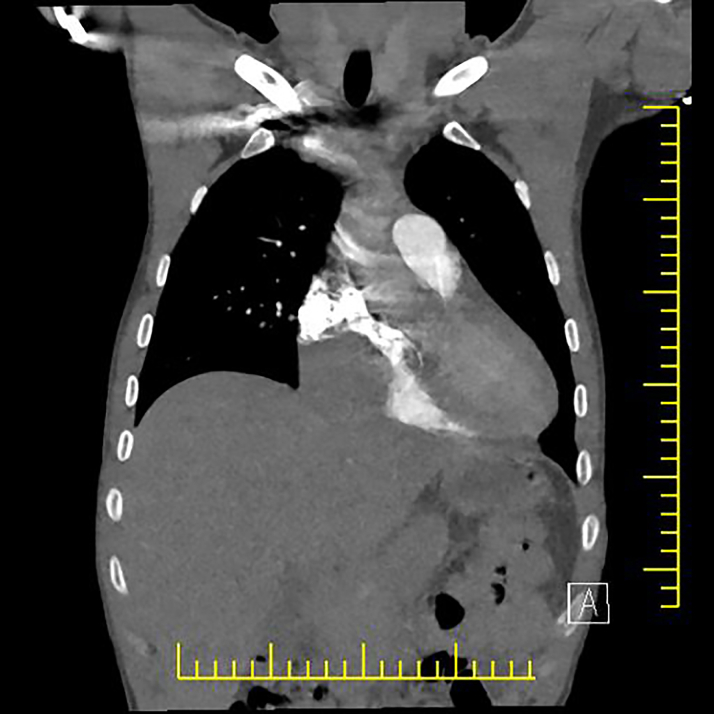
Mass Compressing the Right Ventricle on Pulmonary Computed Tomographic Angiography
